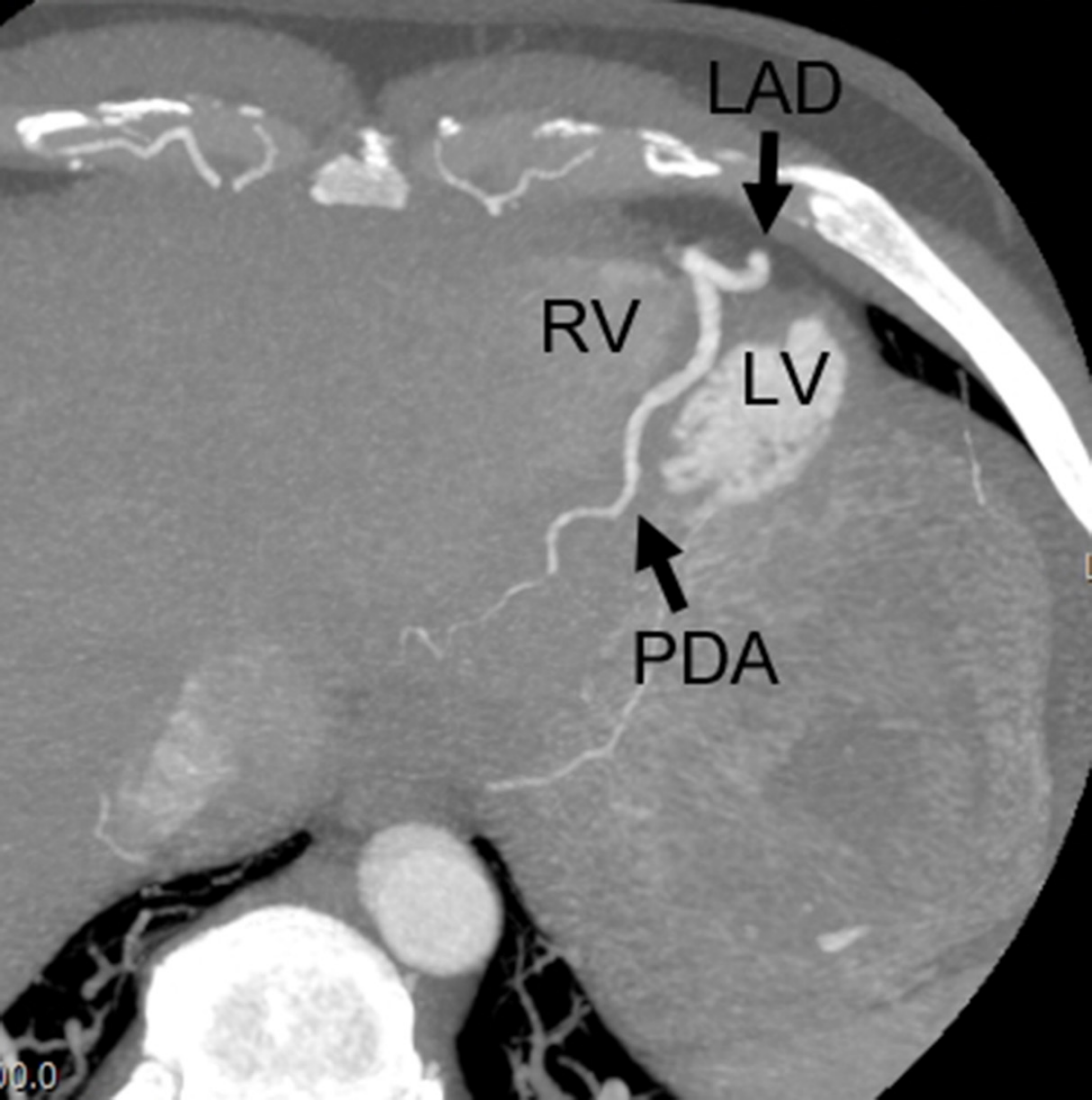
CT coronary angiogram inferior axial maximum intensity projection Figure showing inferior aspect and apex of the heart demonstrating the continuation of the left anterior descending artery (LAD) into the posterior descending artery territory (PDA), between the left ventricle (LV) and right ventricle (RV).
